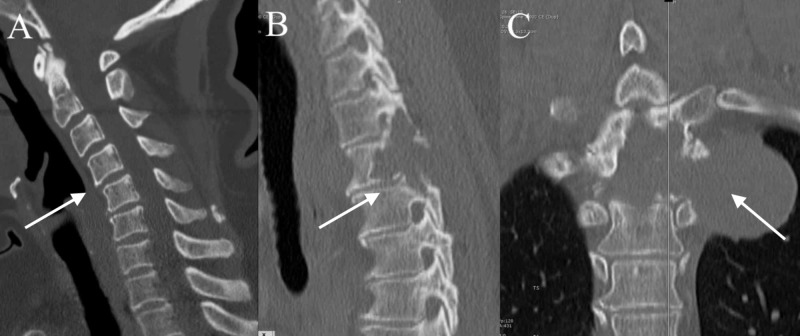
Preoperative CT thorax without contrast Preoperative CT thorax sagittal view without contrast (A) showing cervical kyphosis pronounced at C5. Preoperative CT thorax sagittal view (B) and coronal view (C) demonstrating tumor involvement of the entire T3 pedicle, much of the T3 vertebral body, the ipsilateral T2/3 and T3/4 facet joints, and the superior aspect of the T4 pedicle. CT, computed tomography
